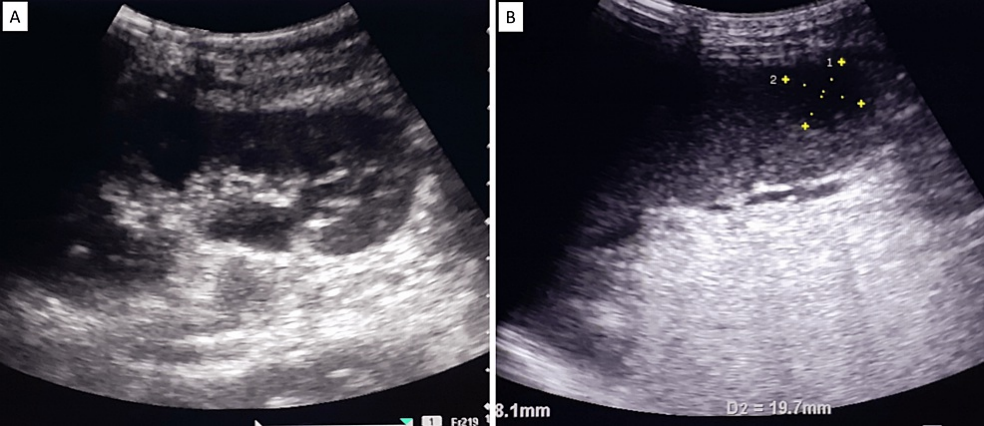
Ultrasound abdomen showing dilated pelvicalyceal system suggestive of hydroureteronephrosis (A) and a hypoechoic lesion with internal echoes in the inferior pole of the spleen suggestive of splenic abscess (B)
